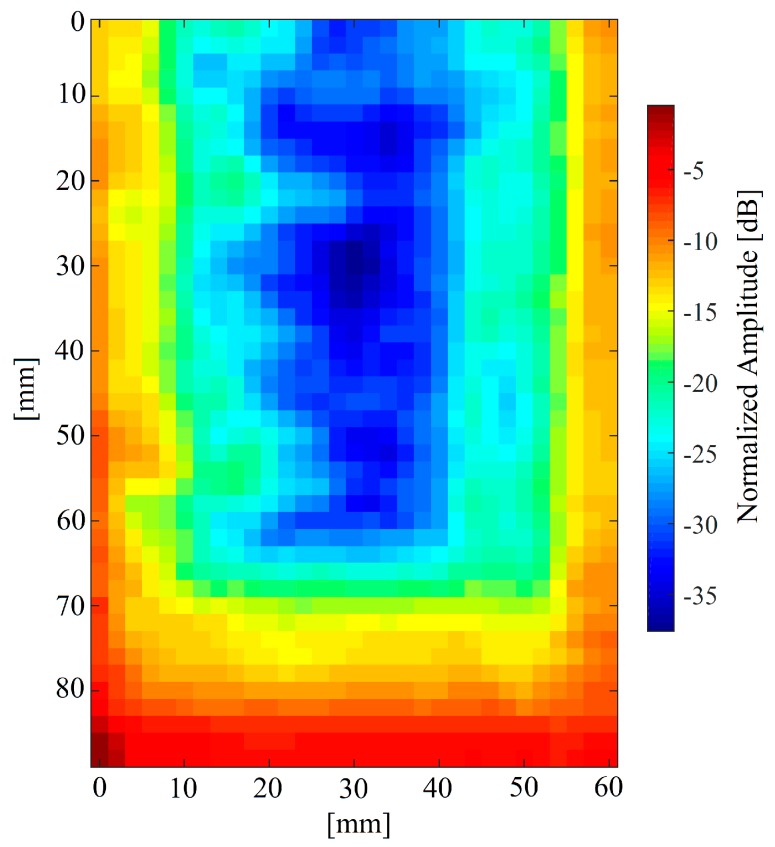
Pulse-compression air coupled ultrasonic results. The image shows the $\tilde{h}\left(t\right)$s normalised amplitude (dB) and the presence of an extended delamination is visible as pixel area with higher attenuation.
